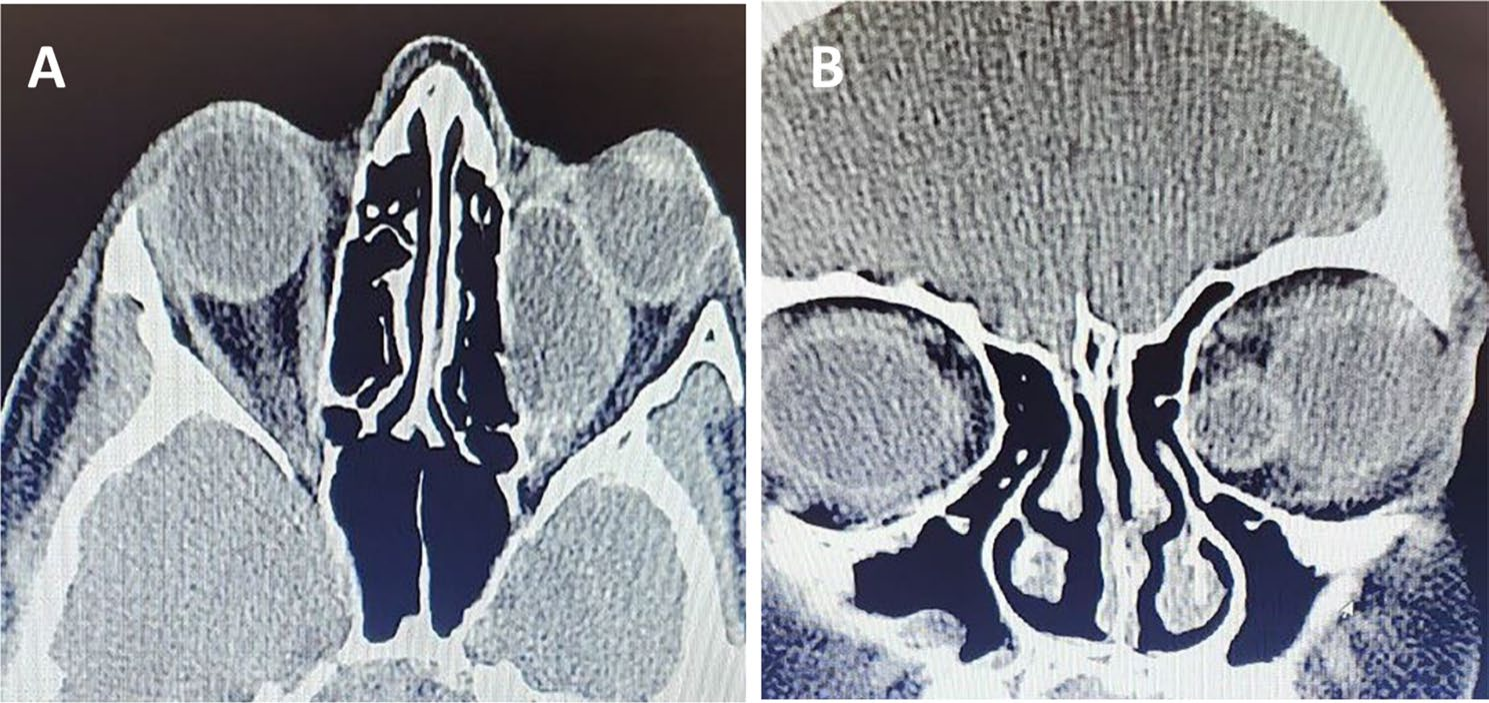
CT scans, axial (A) and coronal (B) orbital CT images revealed a circumscribed, well-defined cystic lesion in the medial rectus muscle
